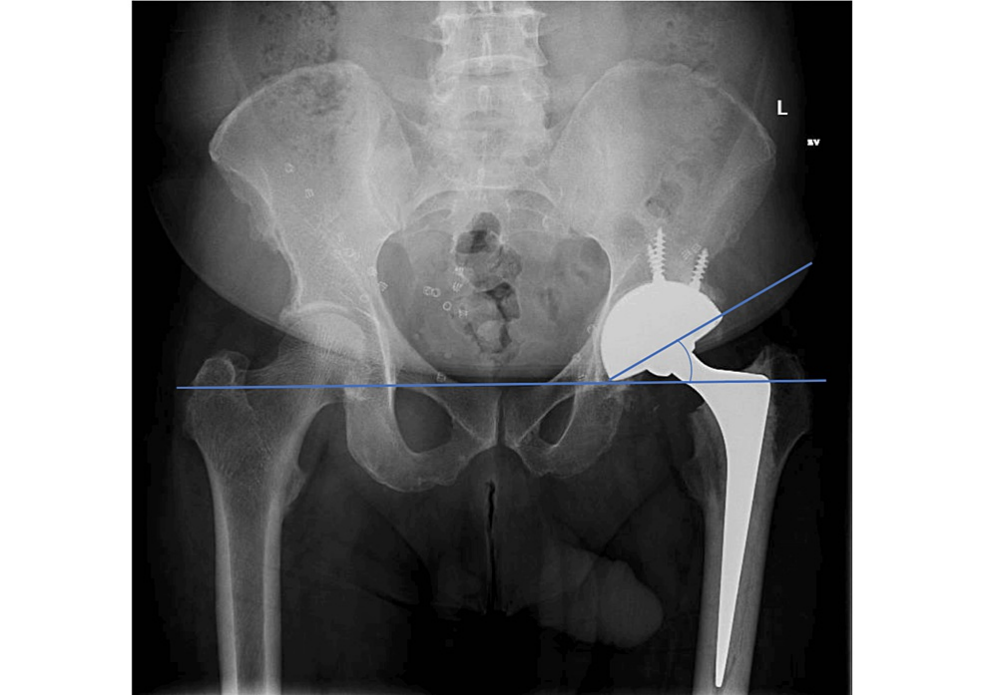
Radiographic cup abduction angle measurement. The cup abduction angle was calculated as the angle formed by the intersection of a line passing through the inferior aspect of the radiographic teardrops and a line tangential to the rim of the cup on pelvic anteroposterior radiographs.
